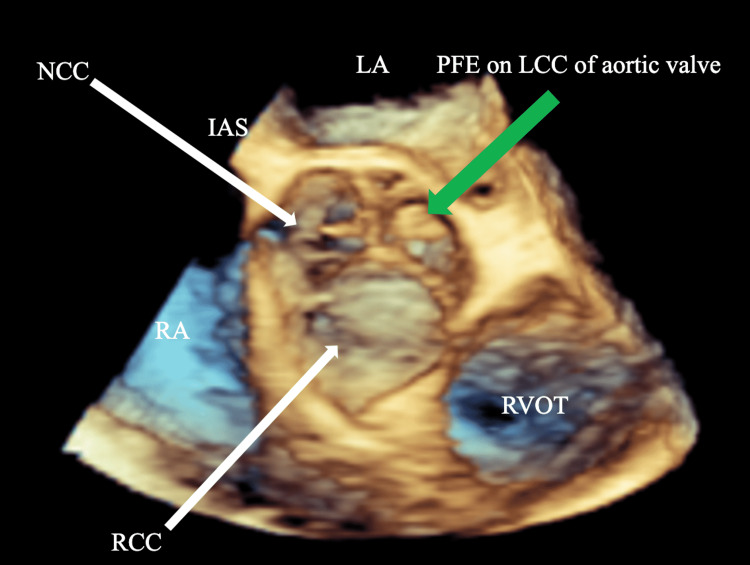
Three-dimensional midesophageal view of the TEE showing the papillary fibroelastoma on the left coronary cusp of the aortic valve (green arrow) RA: right atrium, LA: left atrium, RVOT: right ventricular outflow tract, IAS: inter-atrial septum, RCC: right coronary cusp, LCC: left coronary cusp, NCC: non-coronary cusp, PFE: papillary fibroelastoma, TEE: transesophageal echocardiogram
